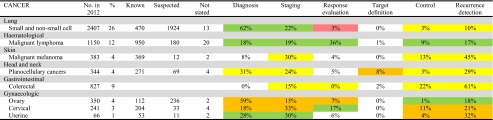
PET/CT scans in the RSD 2012
